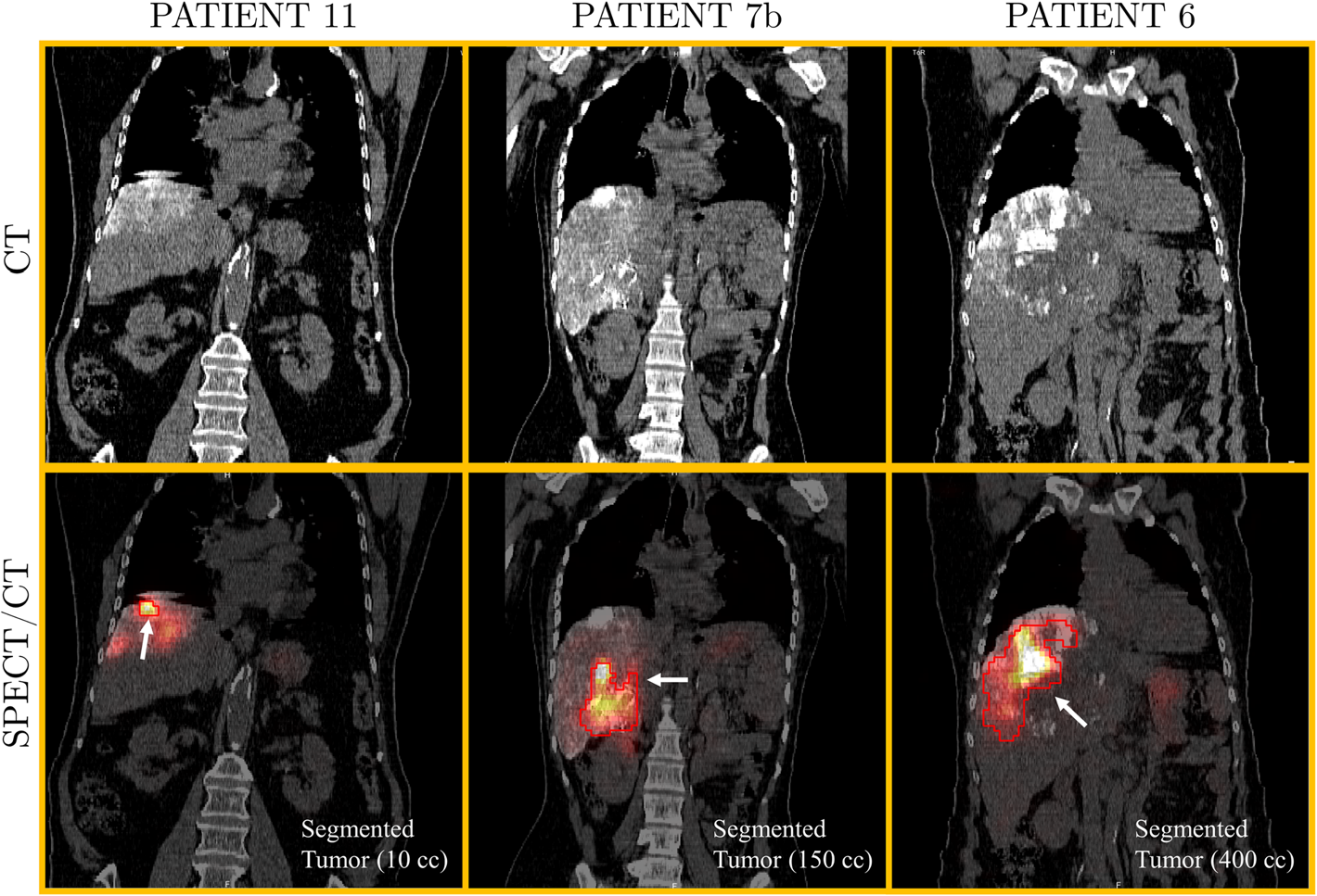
CT and SPECT/CT coronal views of patient no. 11, 7b, and 6 showing the distribution of ^188^Re-HDD-Lipiodol in liver tissue at 3 h after administration of the radiopharmaceutical
